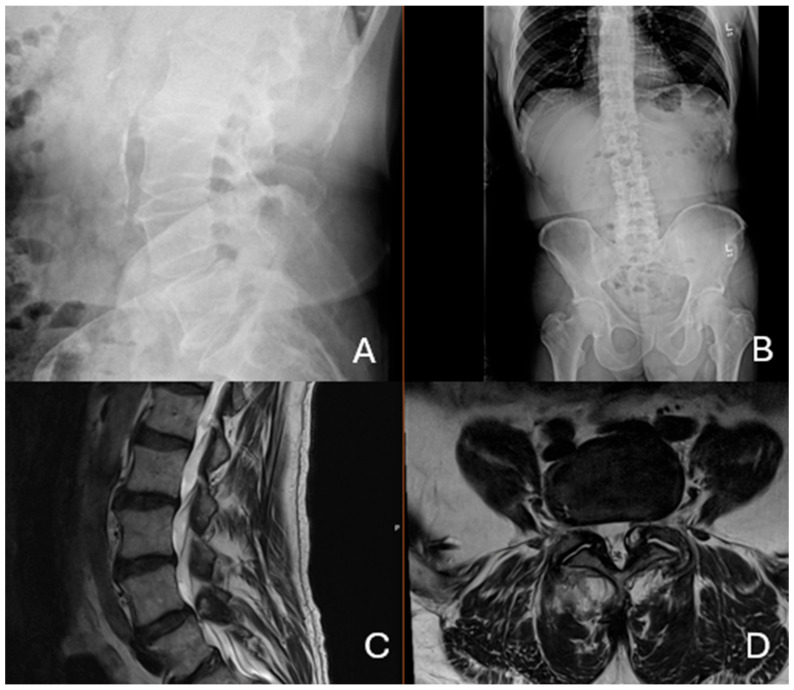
75-year-old male with radiating left leg pain with a synovial facet cyst but without any instability. (A): Preoperative lateral radiograph demonstrating normal sagittal alignment. (B): Preoperative AP radiograph demonstrating normal coronal alignment. (C): Preoperative T2-weight sagittal MRI demonstrating a left-sided synovial facet cyst (D): Preoperative T2-weighted axial MRI demonstrating impingement of the left L5 traversing nerve root due to a compressive synovial facet cyst.
